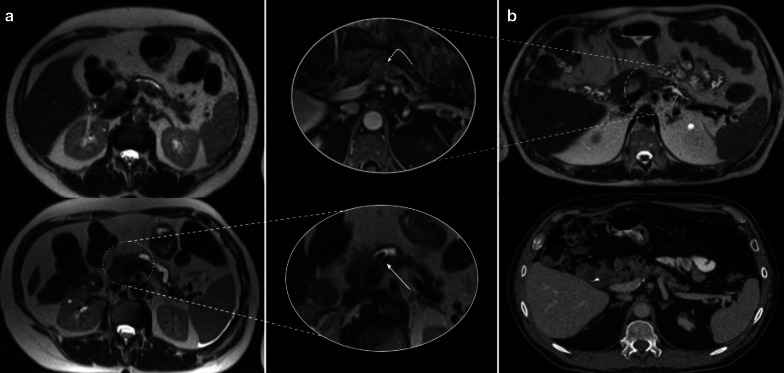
Benign duct dilatation vs. tumor recurrence. (a) 60-year-old female patient during acute abdominal pain. MRI showed pancreatic duct dilatation with filling defect (white straight arrow) just proximal to pancreaticojejunostomy. Acute stump pancreatitis was the final diagnosis. Endoscopic ultrasound confirmed mucoid plug related to pancreaticojejunostomy. Previous MRI (upper) denoted normal wirsung caliber. (b) MRI scan control in a 68-year-old male patient with pancreaticoduodenectomy due to pancreatic cancer. MRI performed three years after surgery reveals increased pancreatic duct dilatation related to an ill-defined hypovascular focal image in the pancreas body (curved arrow in magnified image). Lower: prior CT scan control denotes normal wirsung caliber. Endoscopy ultrasound guided biopsy confirmed adenocarcinoma recurrence
